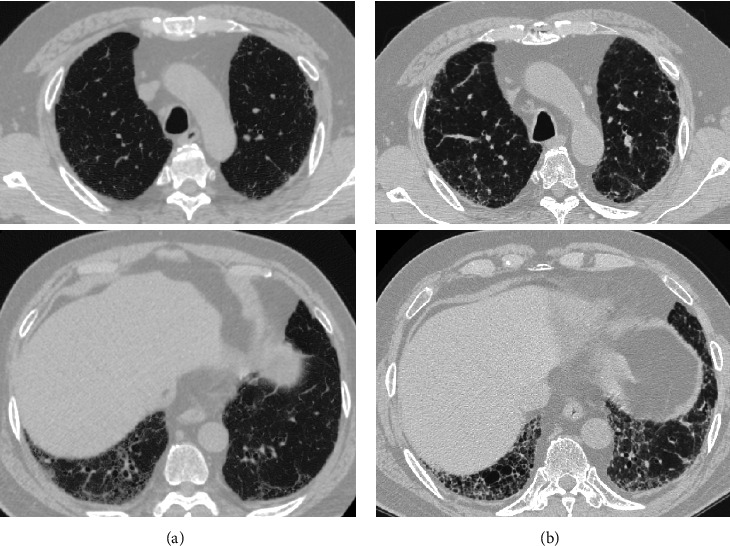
High-resolution CT scans of a representative 62-year-old man sarcoid patient at the time of diagnosis (a) and 3 years after diagnosis (b).
